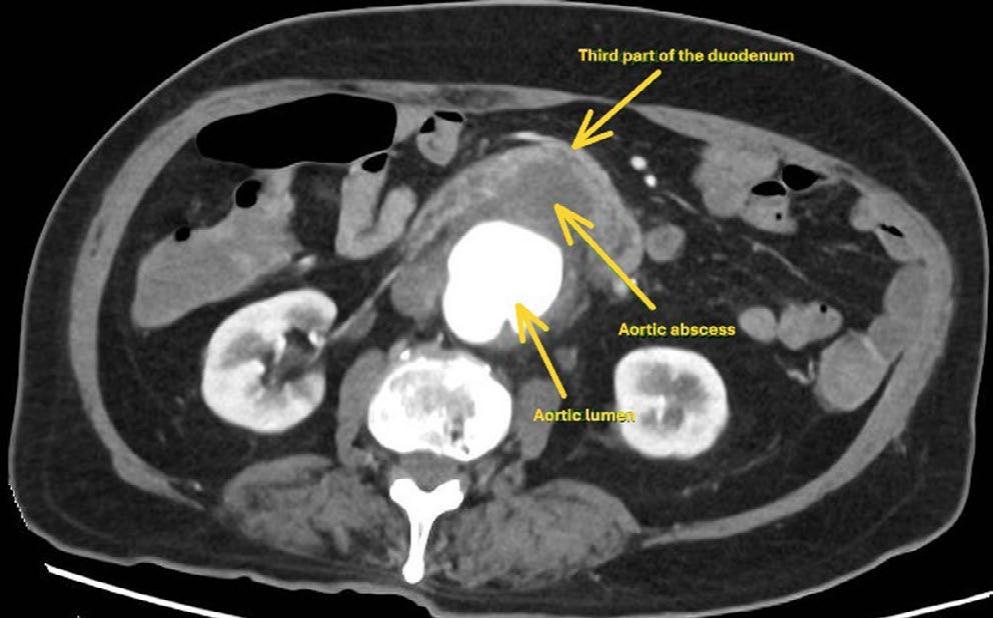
A computed tomography image showing the development of aortic abscess between the abdominal aortic aneurysm and the third part of duodenum.
